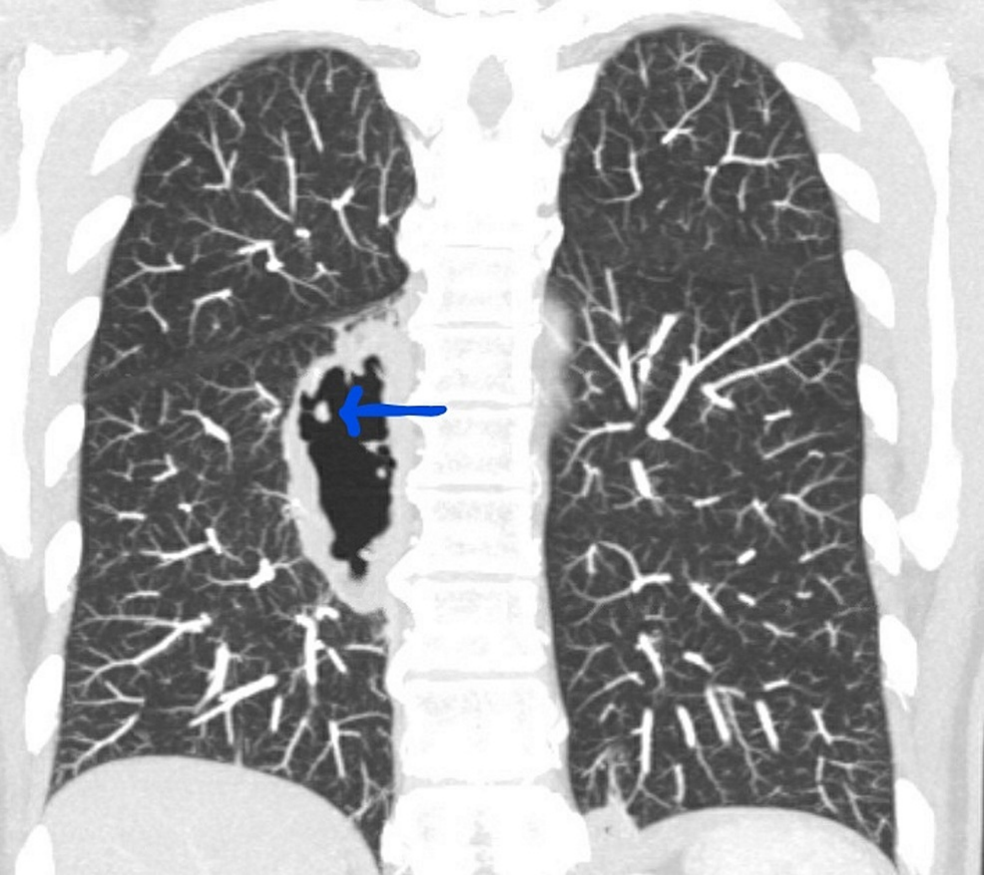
Coronal Reconstruction of Chest Computed Tomography of Case 1 Arrowhead points to cavitary lesion measuring 31 x 78 mm in the right lower lung lobe
